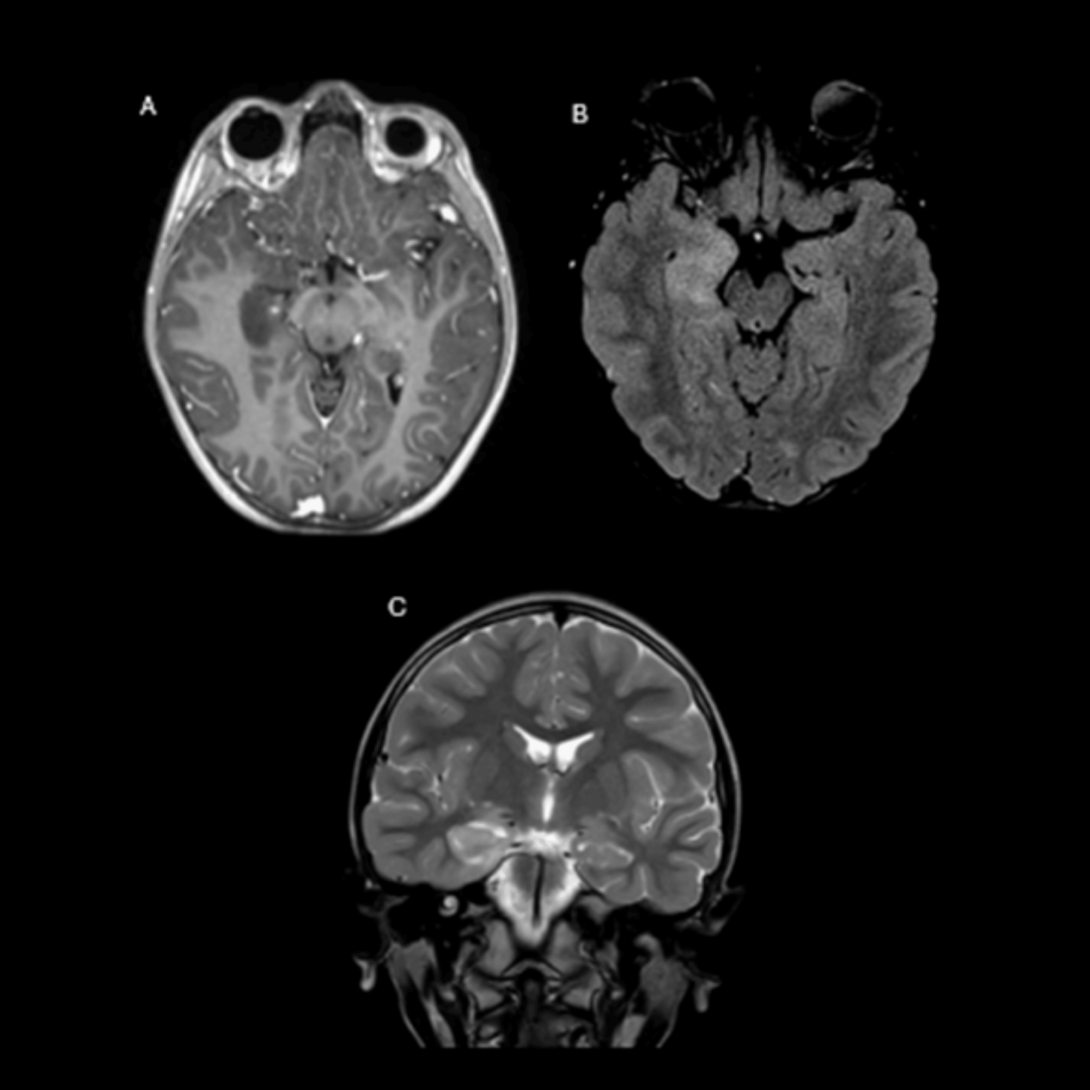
Brain magnetic resonance imaging (A) Axial T1-weighted contrast-enhanced sequence: hypointensity is observed in the right hippocampus with volume enlargement due to edema, without enhancement following paramagnetic contrast administration; (B) Axial fluid-attenuated inversion recovery (FLAIR) sequence: demonstrates diffuse hyperintensity from edema in the mesial temporal cortex, with particular involvement of the right hippocampus and parahippocampal gyrus; (C) Coronal T2 sequence: confirms unilateral involvement of the right mesial temporal structures, with no contralateral involvement.
